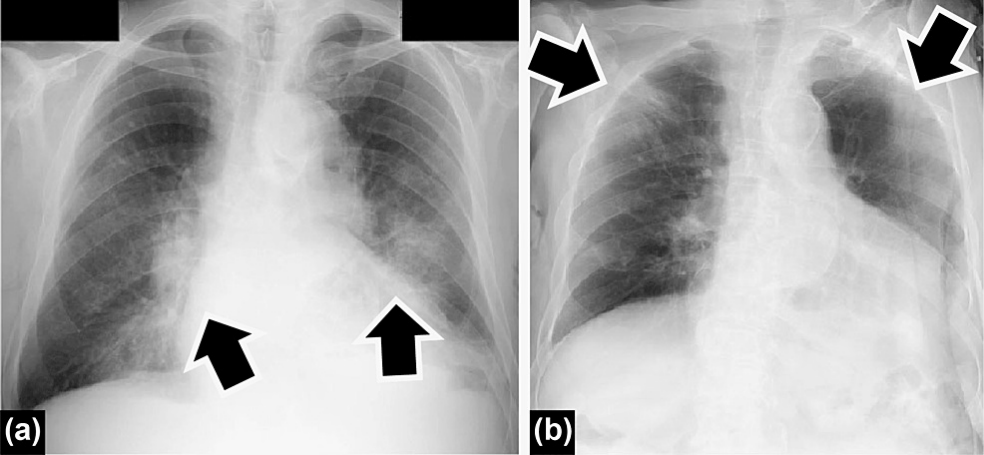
Chest radiography images of the patient in the second case (a) At revisit. (b) At the general ward.
